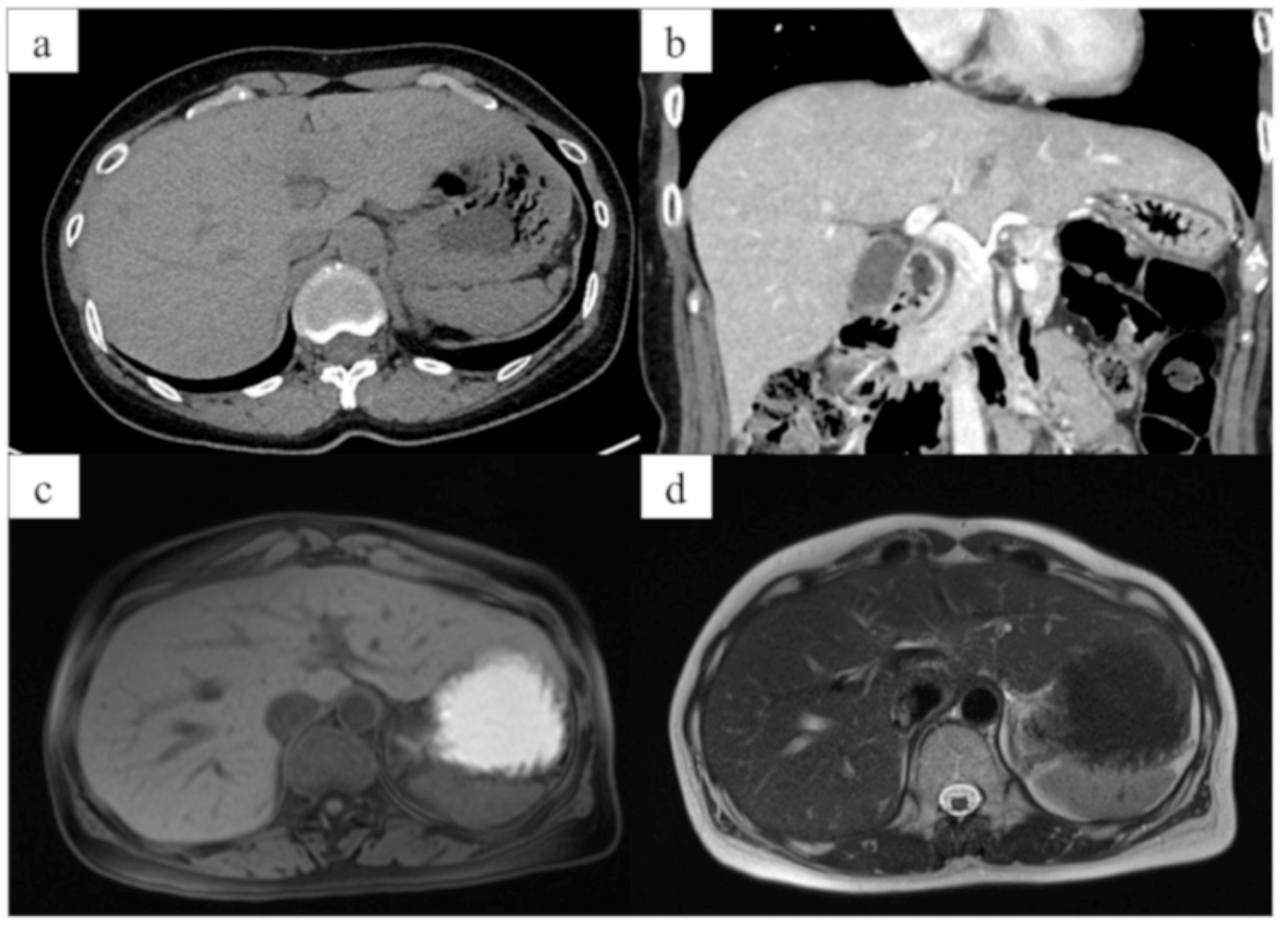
Imaging of a living liver donor candidate a: Unenhanced computed tomography (CT) showed no evidence of hepatic steatosis. b: Contrast-enhanced CT revealed no remarkable abnormalities. c: No significant abnormalities were observed on T1-weighted magnetic resonance imaging (MRI). d: No significant abnormalities were observed on T2-weighted magnetic MRI. CT and MRI showed no evidence of hepatic steatosis or structural abnormalities.
